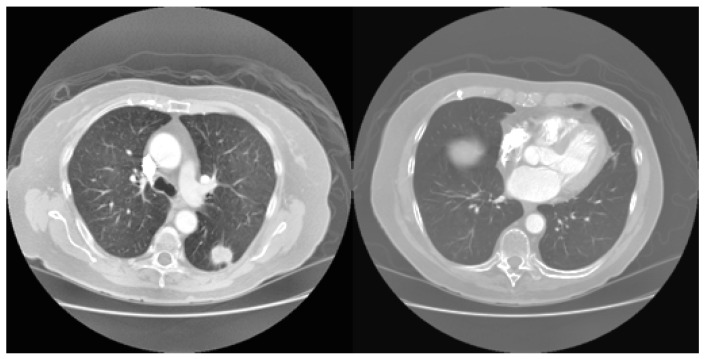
Sample CT images considered for experimenting with the SHOA-DNN model.
